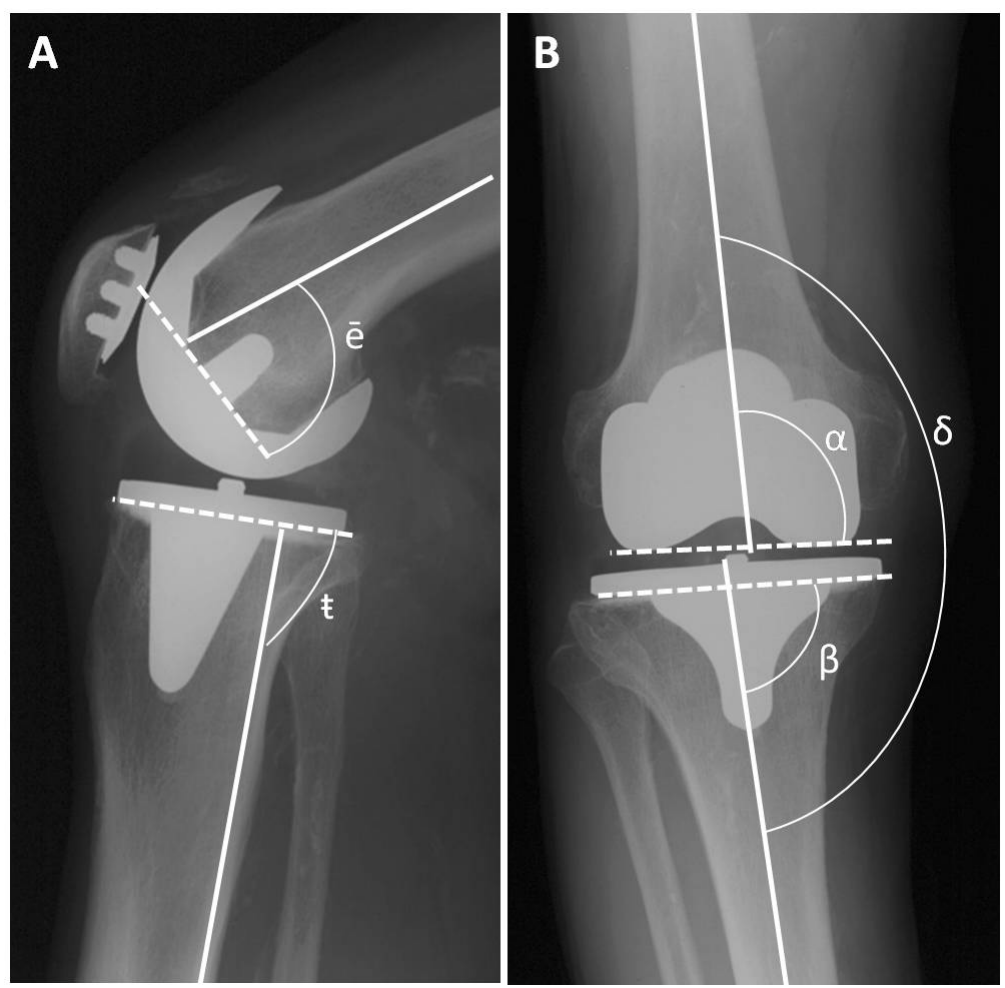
Surgical accuracy on long leg radiographs. Component positioning angles were measured on standing long leg radiographs with lateral (A) and antero-posterior-view (B). Angles between component axis (broken line) and mid-tube bone axis (straight line) were measured for tibia component slope (g), femur component flexion-extension (ē), femur component varus-valgus (α) and tibia component varus-valgus (β). Tibiofemoral varus-valgus (δ) was measured between long bone axes. For slope measurements (g), the posterior cortex line served as reference.
